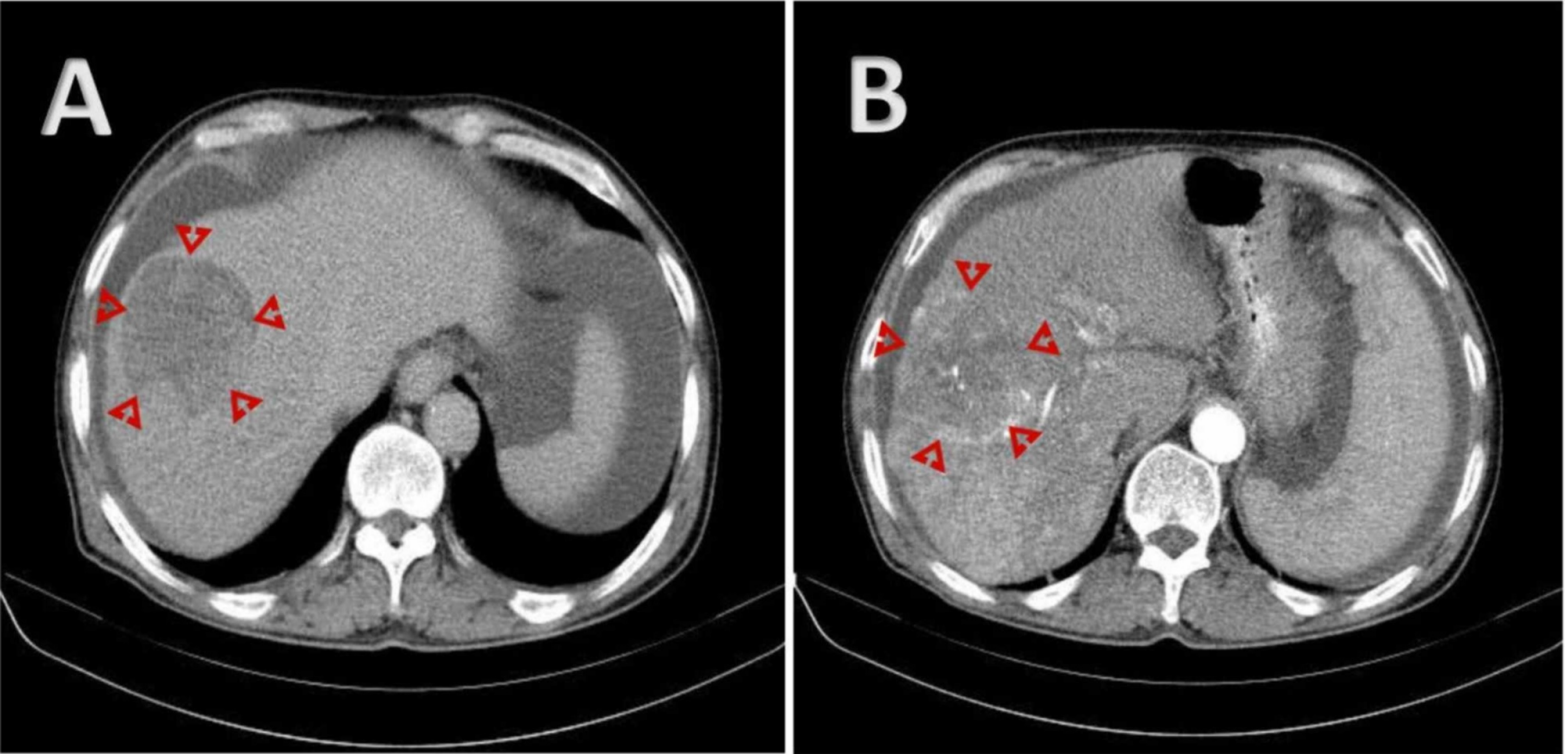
(A-B) CT imaging showing a mass in a cirrhotic liver with mosaic enhancement on early phases and rapid washout on delayed phase, compatible with hepatocellular carcinoma A: Axial C + arterial phase; B: Axial C + portal venous phase Image courtesy of Dr Mohammad Taghi Niknejad, Radiopaedia.org, rID: 20886; https://radiopaedia.org/cases/hepatocellular-carcinoma-with-portal-vein-tumour-thrombosis
